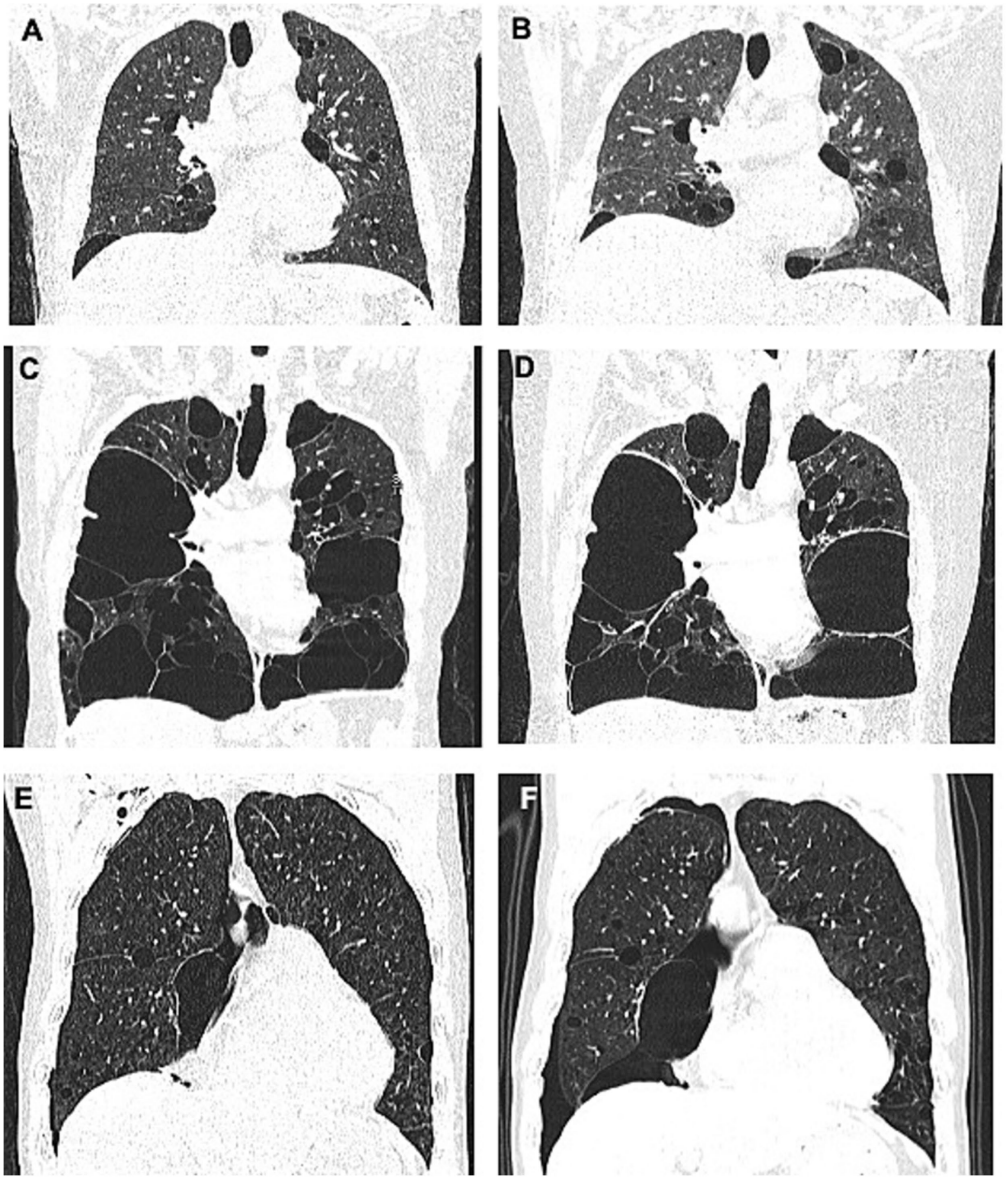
(A,B) represent the lung CT results of the proband in Family 2 in May 2019 and January 2024, respectively. (C,D) represent the lung CT results of the proband in Family 4 in August 2017 and May 2024, respectively. (E,F) represent the lung CT results of the proband in Family 5 in November 2023 and August 2024, respectively.
